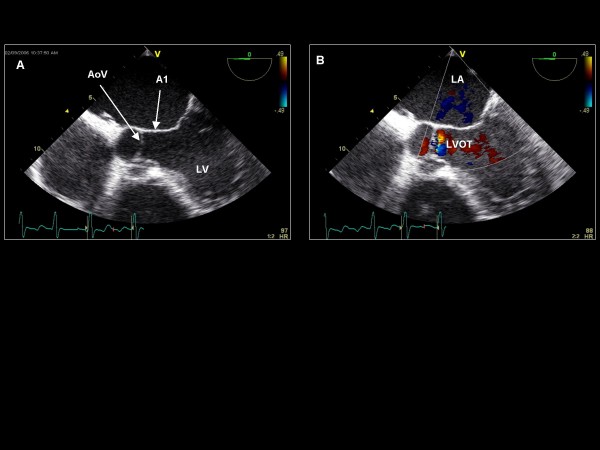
Imaging plane 0°, (A) The aortic valve (AoV) and A1 segment of the mitral valve. (B) Color Doppler applied to the aortic valve demonstrating trivial aortic regurgitation within the LVOT.
